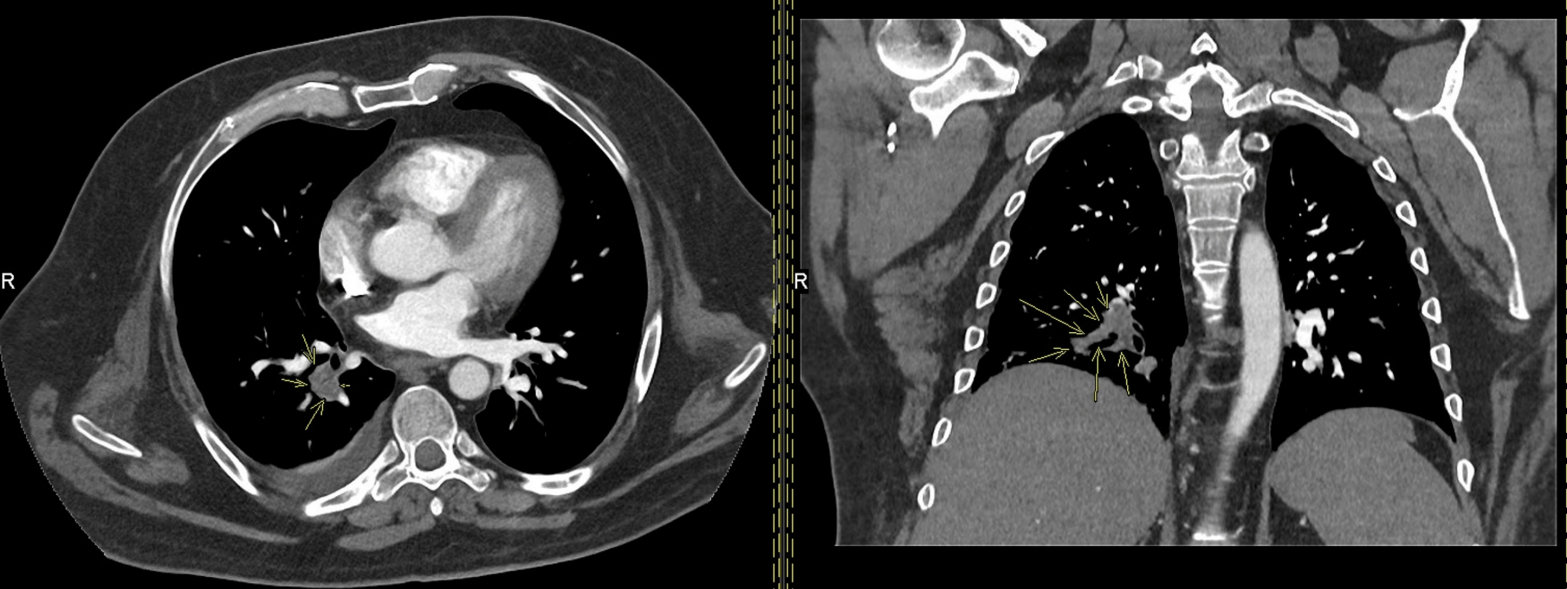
CT pulmonary angiography revealing filling defects (yellow arrows) in multiple segmental and subsegmental branches on the right side.
